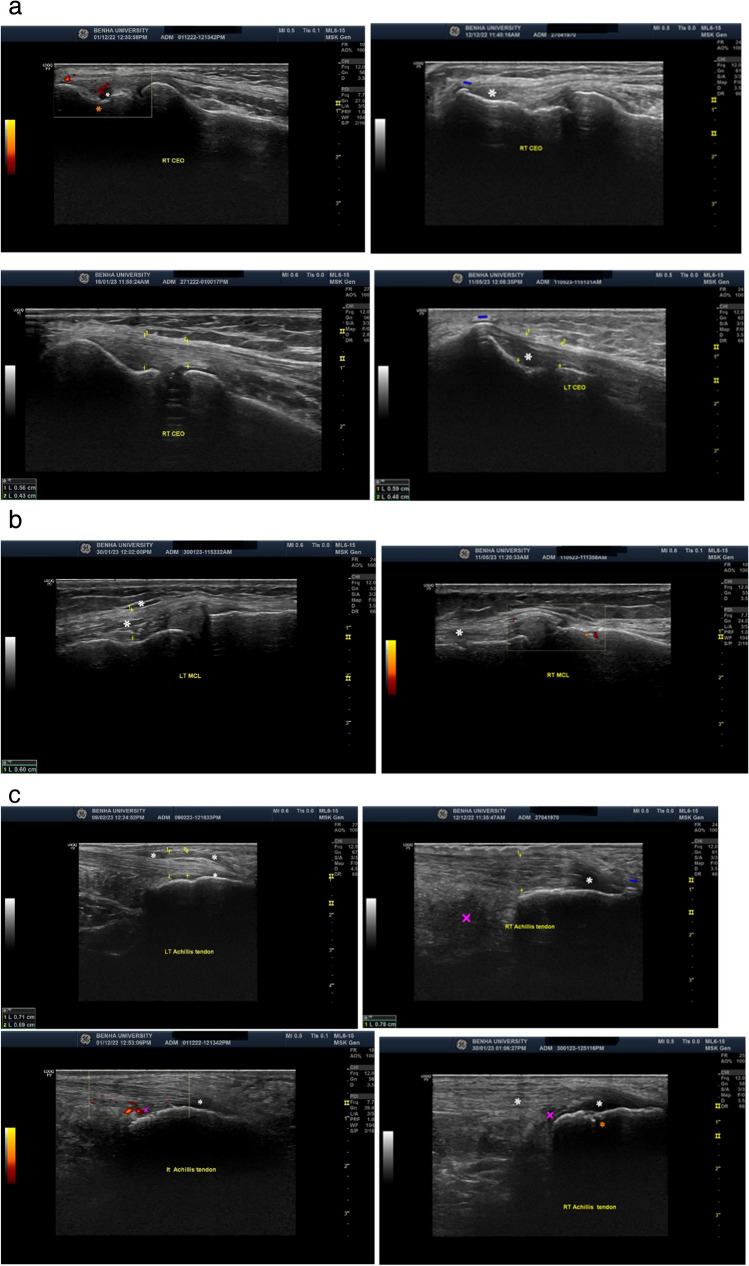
MSUS findings at sites of LEI entheses. a CEO enthesopathy in the form of increased thickness, soft tissue edema, presence of enthesophyte, erosion, and active Doppler signals. b MCL enthesopathy in the form of increased thickness, soft tissue edema, and erosion with an active Doppler signal. c Achilles tendon enthesopathy in the form of increased thickness, soft tissue edema, presence of enthesophyte, active Doppler bursitis, and erosion. White asterisk (*): hypoechoic area and soft tissue edema; orange asterisk (*): erosion; blue MDASH (–): enthesophyte; violet cross (×): bursa; yellow plus sign (+): thickness
